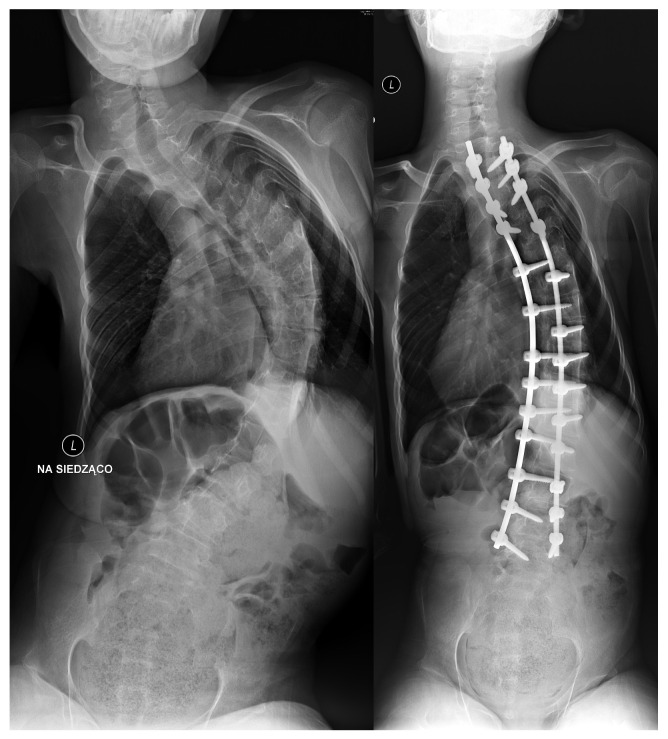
X-ray of the spine before and after surgical correction of scoliosis. A 13-year-old boy with thoracolumbar scoliosis. AP X-ray before and after surgery [own source].
